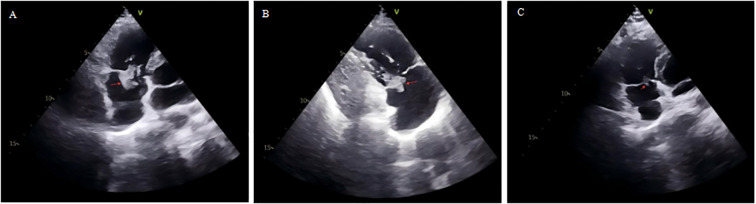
Serial echocardiographic images showing regression of the tricuspid vegetation. (A) At baseline: a large vegetation measuring 24.1 × 19.3 mm. (B) After one month of treatment: the vegetation decreased to 12.0 × 12.3 mm. (C) After nine months of treatment: the vegetation regressed to 7.9 × 3.0 mm with preserved valve function.
